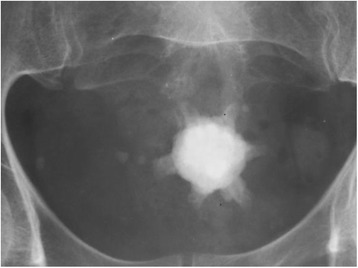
XRay KUB showing a irregular shaped stone
